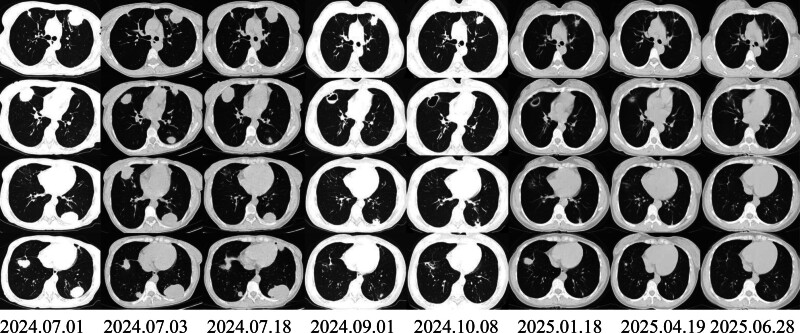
High-resolution CT of the patient’s lungs at the early stage of the disease. Multiple nodules of varying sizes can be seen in both lungs, some of which are round or nearly round, while others are irregular, with mixed density, mainly low density, and have shallow lobulation and spiculation. Some of them are fused. With the progress of treatment, the multiple mixed-density nodules and nodules in both lungs have significantly decreased in size and number. No cavities are shown in the lesions. Currently, multiple solid patchy and fibrous cord-like shadows are prominent in the local areas. CT = computed tomography.
